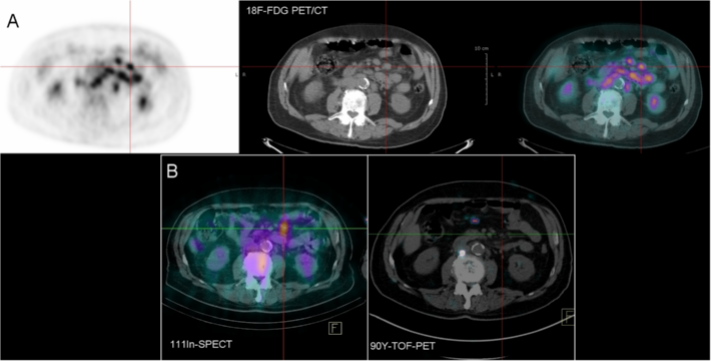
^18 ^F-FDG PET and immuno-PET. Patient with follicular lymphoma injected with ^111^In- and ^90^Y-Zevalin® (Bayer) 7 days apart. (A) Pre-therapeutic scan after injection with ^18^ F-FDG and (B) immunoscintigraphy recorded 6 days (bottom left) after ^111^In-Zevalin® (Bayer) injection showed an abnormal focus corresponding to a lymphoma mass detected on the pre-RAIT CT and ^18^ F-FDG PET (A). No relevant signal was detected by ^90^Y-PET (bottom right).
